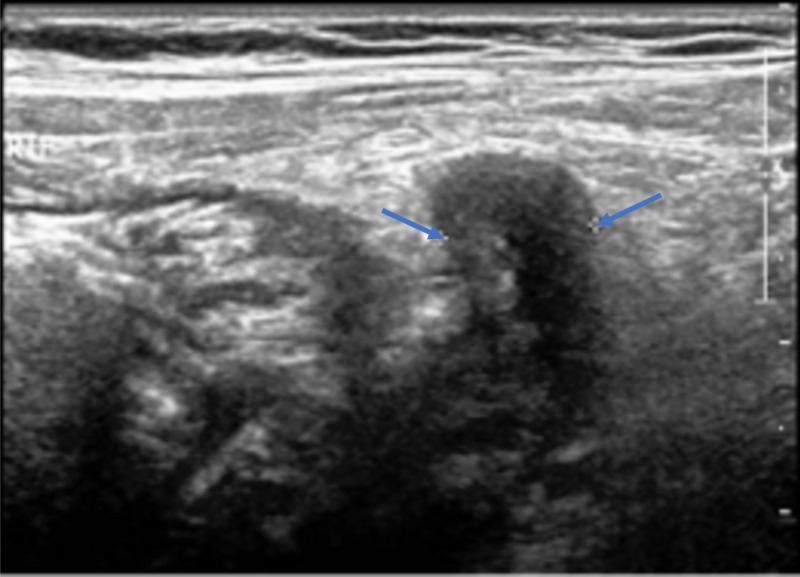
Ultrasonography of the abdomen - view 1 The image shows noncompressibility and discontinuity in the appendicular wall, with a maximum diameter of 0.9 cm (arrows)
